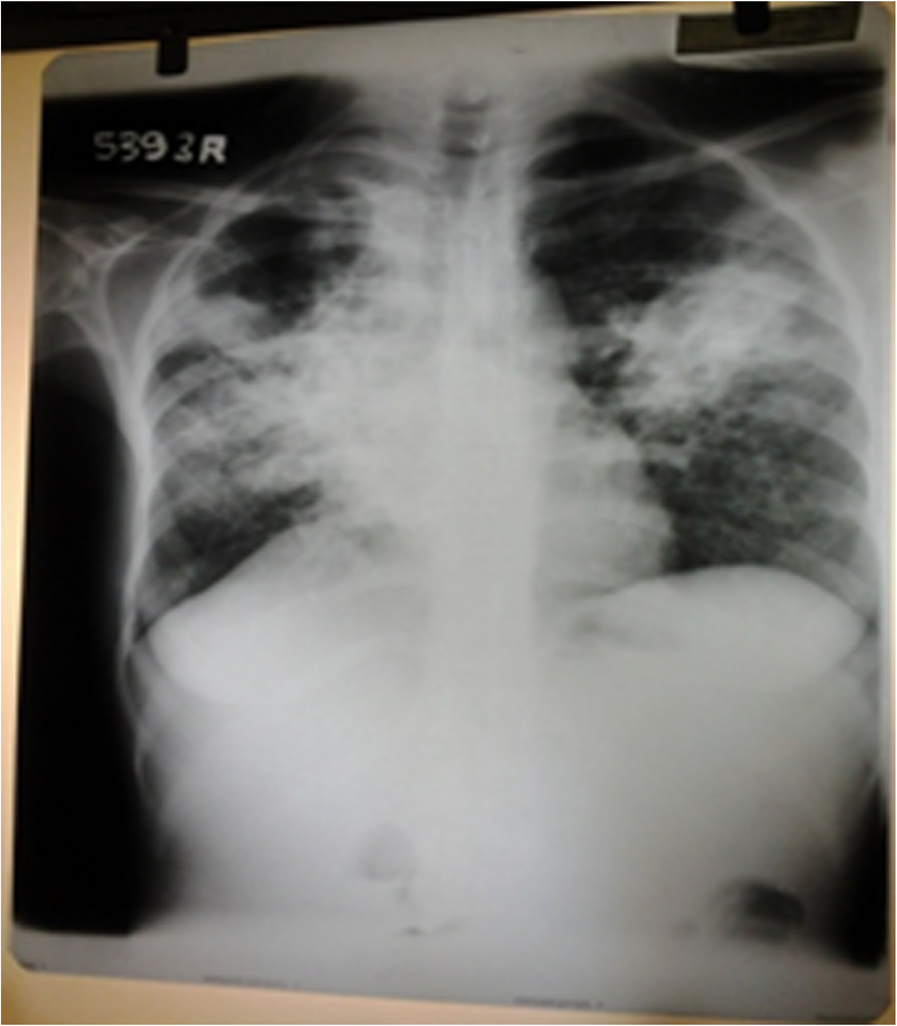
(Chest x-ray on Day 1 of admission). Opacification predominantly involving the right mid zones. There are adjacent nodular opacities with large cavitatory lesion at the right upper zone. Right heart border is obliterated. Mediastinum has shifted to right. No pleural effusions or bony erosions.
